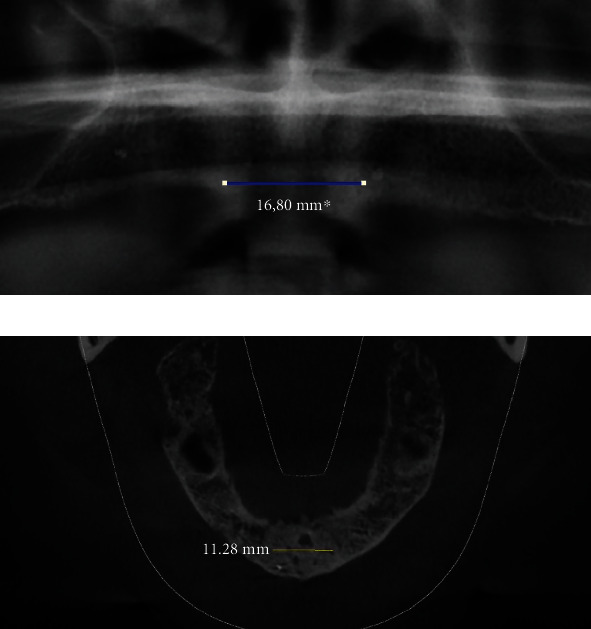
Measurement of the shortest vertical distance between the right and left maxillary lateral incisor tooth region on a panoramic image (a) and a cone beam computed tomography image (b).
